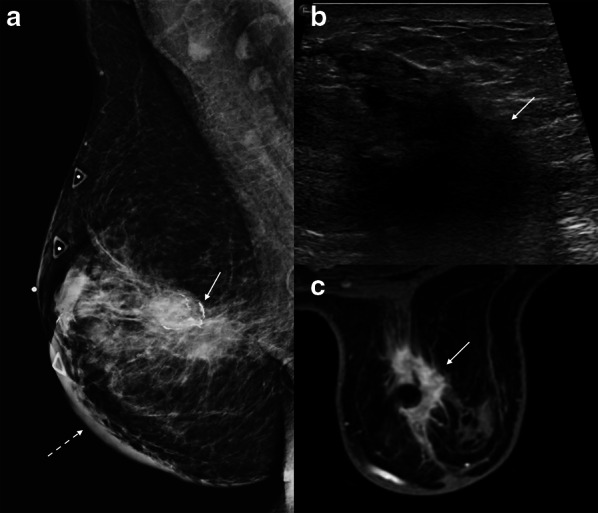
Fat necrosis. A 52-year-old woman with remote history of mastopexy presented with a palpable breast mass. Mediolateral oblique mammogram (a) shows an irregular mass with spiculated margins, associated coarse rim calcifications (solid arrow), and focal skin thickening (dashed arrow). Longitudinal grayscale ultrasound (b) shows a non-parallel irregular hypoechoic mass (arrow). Fat-suppressed axial post-contrast T1-weighted breast MRI (c) shows an irregular mass with spiculated margins (arrow) with associated singal void artifact related to post-biopsy clip marker. Ultrasound-guided biopsy showed fat necrosis. Repeat biopsy under MRI guidance confirmed the diagnosis of fat necrosis
